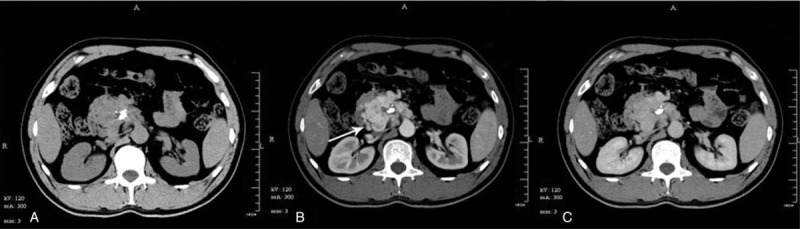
(A) Routine abdominal CT showing an isodense mass in the pancreatic head relative to the liver density, with lobulated margins and the presence of calcification. (B) Contrast-enhanced abdominal CT showing severe heterogeneous enhancement of the mass, with a clear boundary and multiple tortuous blood vessels in the arterial phase observed peripherally (arrow). (C) Slightly decreased venous phase enhancement of the mass, which still appears relatively hyperdense.
